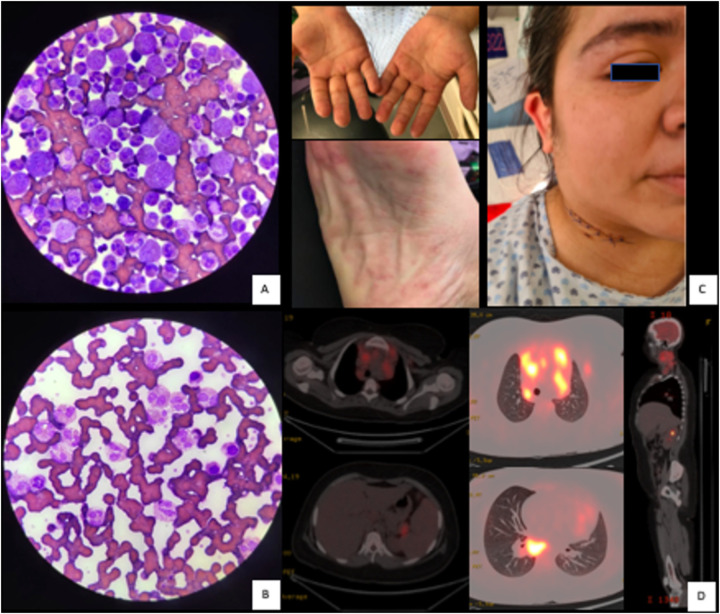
Marked eosinophilia in bone marrow aspirate (A) and peripheral blood smear (B), clinical presentation showing extensive cervical lymphadenopathy and palmoplantar erythema (C), and PET-CT scan demonstrating supra- and infradiaphragmatic tumor activity with splenic involvement, consistent with an incomplete metabolic response (Deauville score 4) (D).
